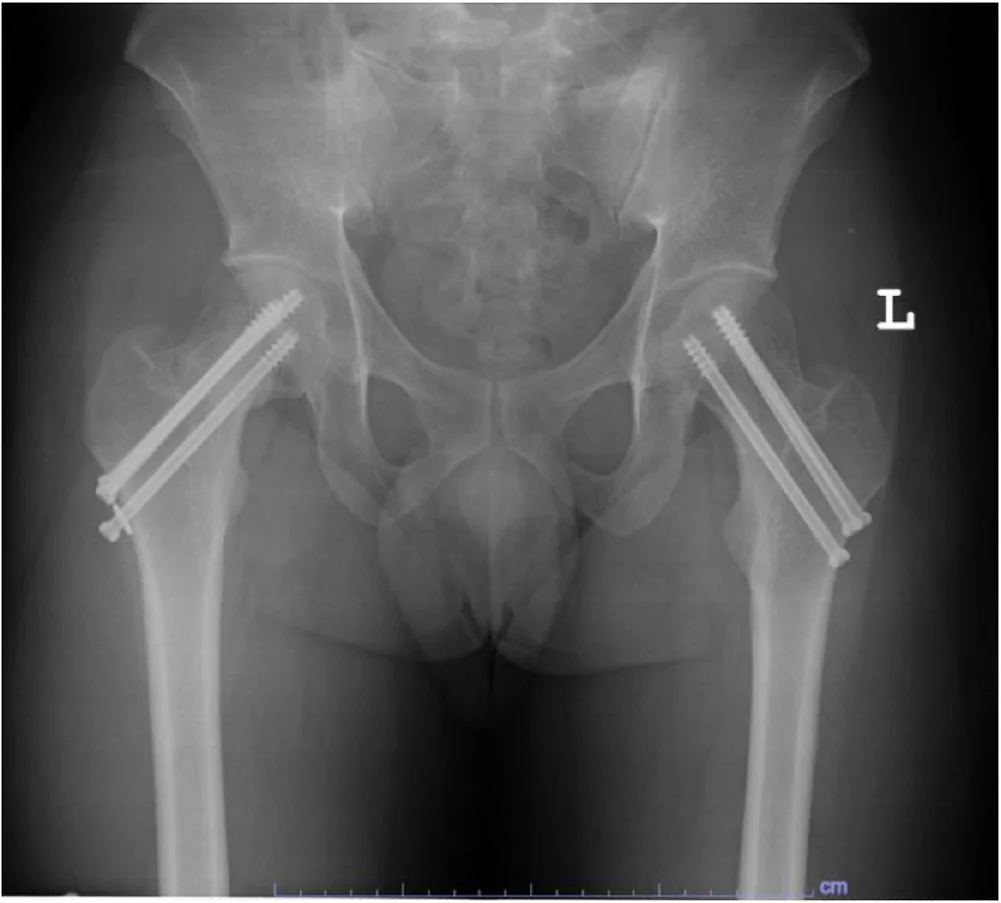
Pelvis AP radiograph showing fracture union over bilateral neck of femur.
